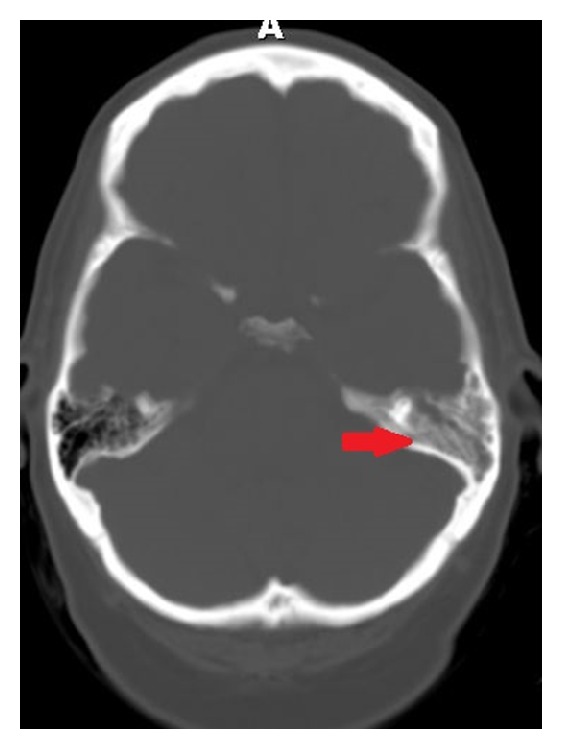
The CT scan shows nonspecific density in the temporal bone without bony erosion (red arrow).
